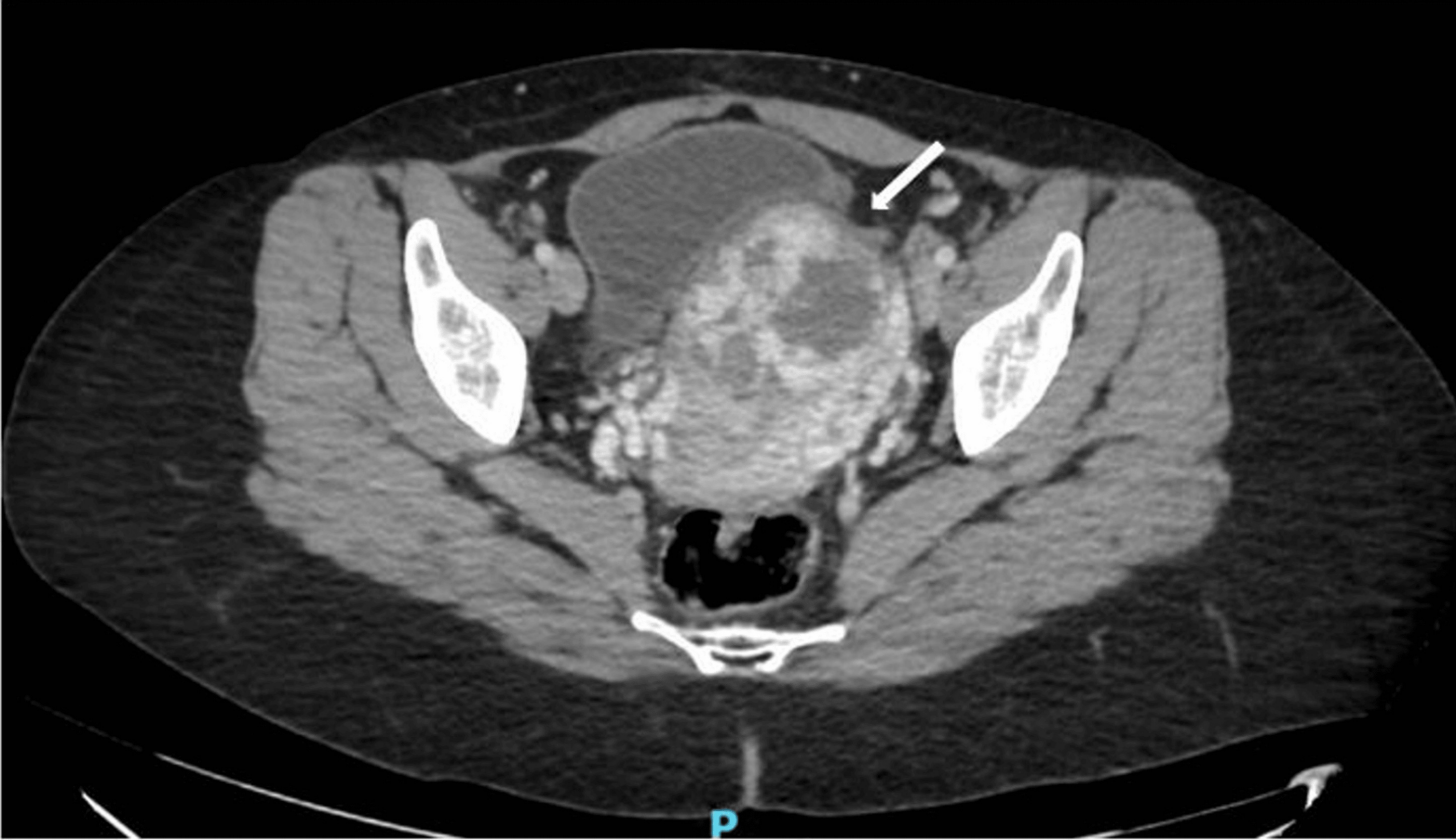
CT of the pelvis accentuates the low-lying nature of cesarean scar ectopic pregnancy and affirms the previously suspected imaging findings of a molar pregnancy. The arrow highlights the thin myometrium on the anterior uterus, raising concern for invasion into local structures and an elevated risk of uterine perforation, bladder injury, and hemorrhage if dilation and curettage were performed as the primary treatment modality.
